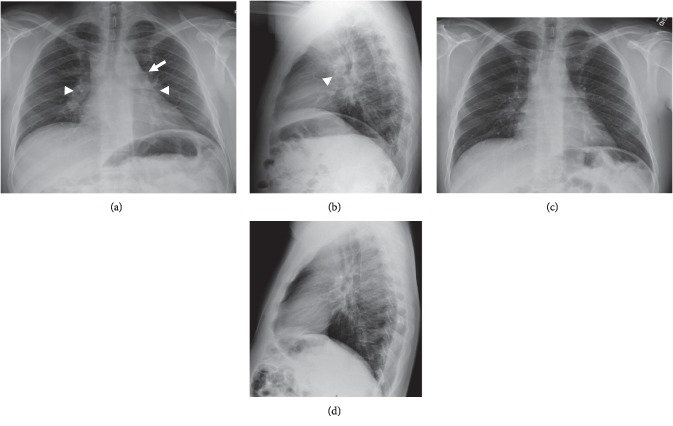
PA and lateral chest radiographs obtained 5 years earlier (a, b) show mild convexity at the AP window (arrow) and subtle perihilar fullness (arrowheads) which in retrospect may have corresponded to lymphadenopathy rather than enlarged pulmonary arteries as initially interpreted. However, chest radiographs at current presentation (c, d) show no evidence of pulmonary or mediastinal disease.
